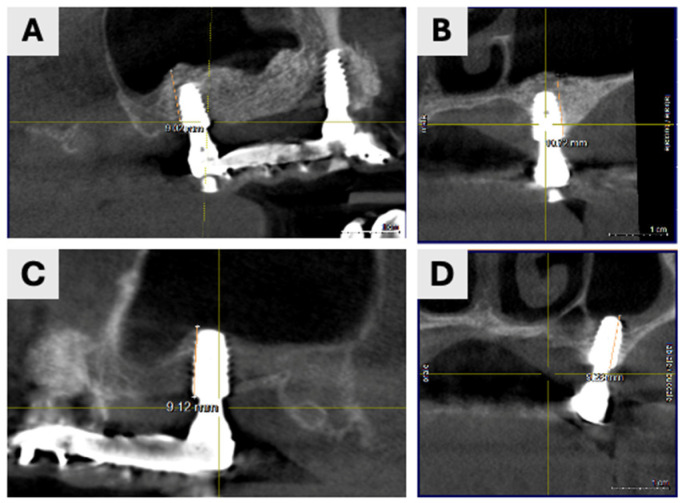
Radiological assessment at the two-year follow-up. (A) Sagittal CBCT view of implant site 16, demonstrating a vertical bone height of 9.02 mm with persistent radiopaque graft material surrounding the implant apex. (B) Coronal CBCT view of the same implant site (16), showing a vertical bone height of 10.72 mm. (C) Sagittal CBCT view of implant site 26, demonstrating a vertical bone height of 9.12 mm and mature native bone surrounding the implant. (D) Coronal CBCT view of the same site (26), showing a vertical bone height of 9.28 mm, with stable peri-implant bone and no radiographic signs of pathology or radiolucency.
